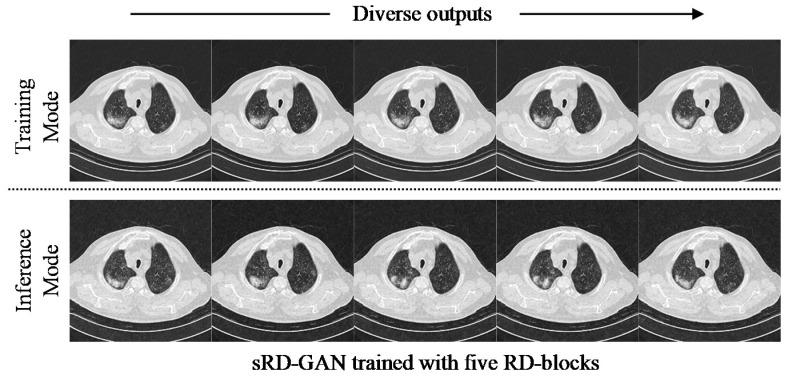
Synthetic COVID-19 CT images generated in training and inference modes, where the diverse outputs generated in inference mode show more apparent differences between the synthetic instances.
